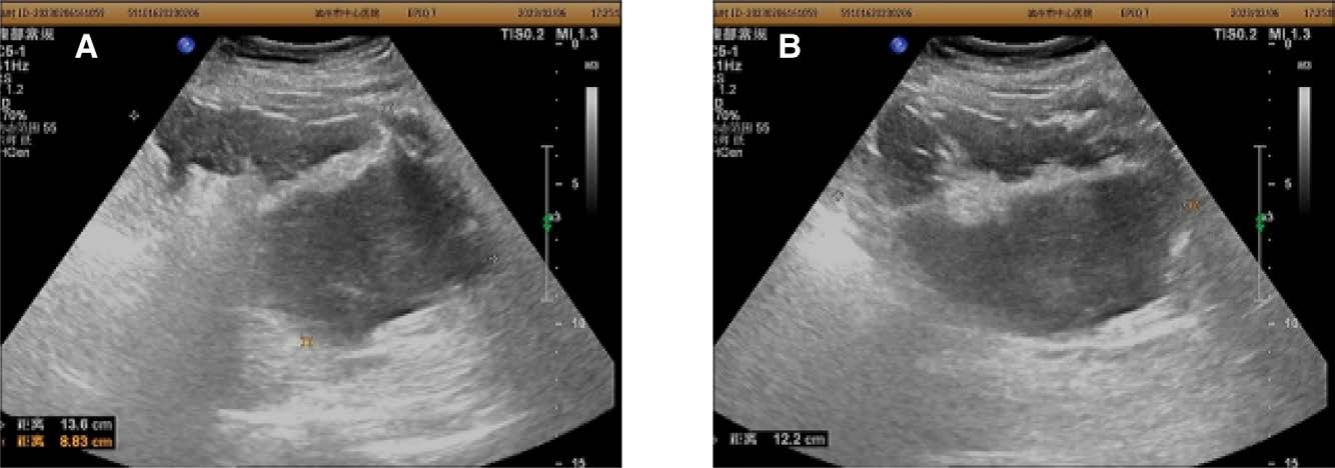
(A and B)Transabdominal ultrasonography showed a mixed echogenic masse measuring 12.2 × 13.6 × 8.3 cm in lower abdominal.
